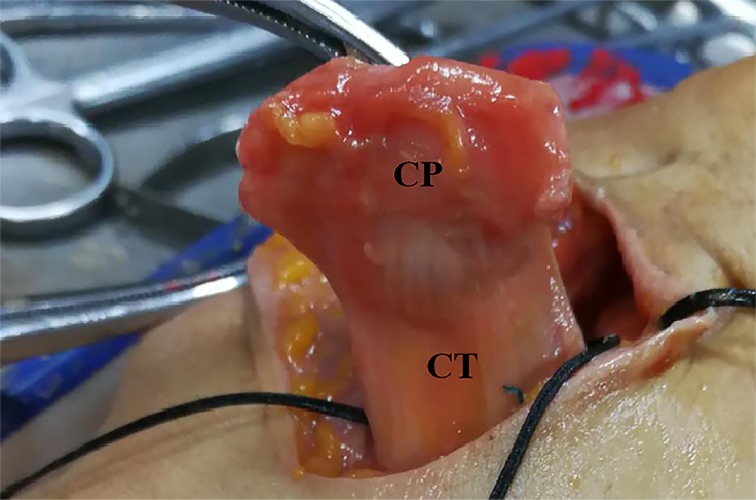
The free bone side of the CP and CT after Latarjet osteotomy procedure
